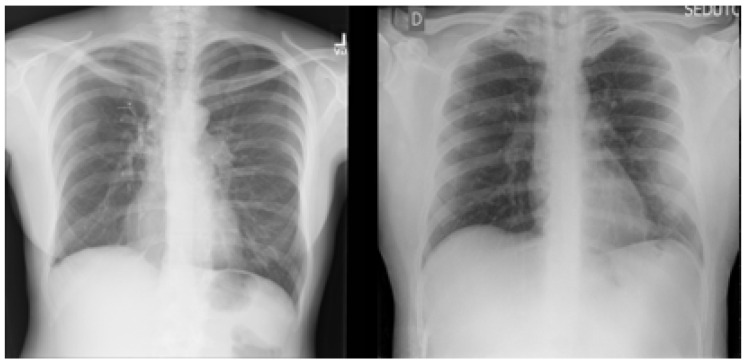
X-ray images of Normal and Abnormal.
